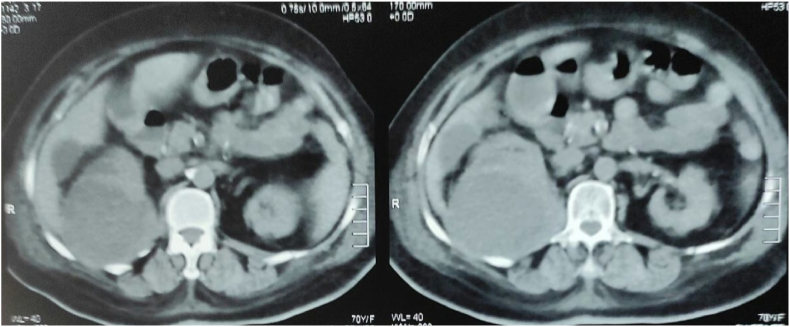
Computed tomography showes right perirenal abscess.
